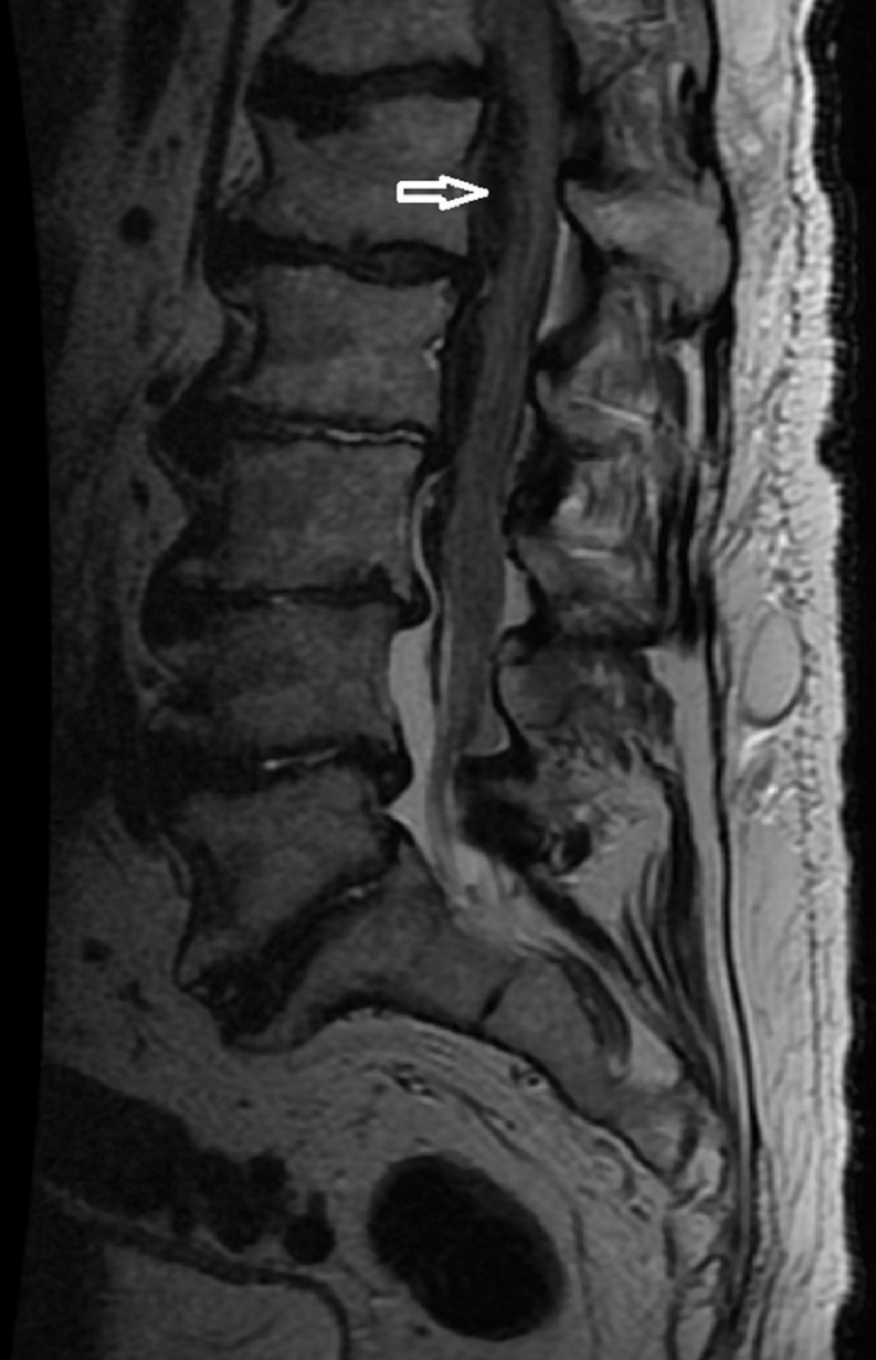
MRI Lumbar Spine T2 Weighted White arrow pointing to hematoma.
